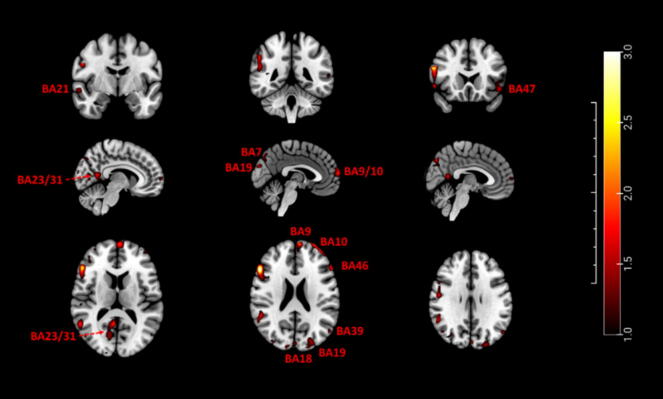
Brain activation maps during the controlled recall of RED/authentic NDE memories, as measured by functional MRI. The figure displays sagittal, coronal, and axial sections averaged across participants, highlighting the most significantly activated regions (pFWE < 0.05). Note: In the coronal and axial slices, the left side of the image corresponds to the patient's right hemisphere, following radiological convention. (For interpretation of the references to color in this figure legend, the reader is referred to the web version of this article.)
